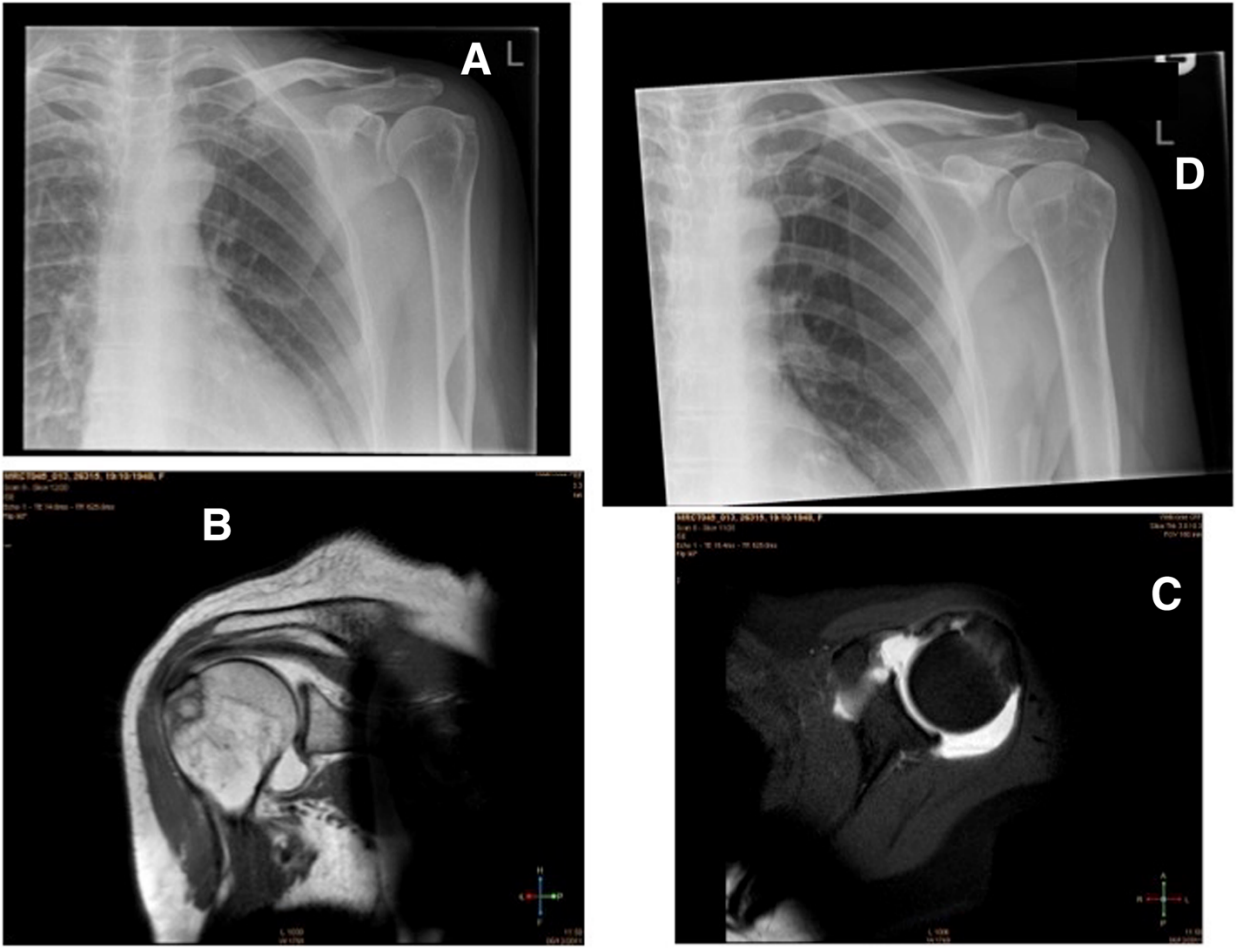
a Left shoulder Plain radiograph coronal plane reported as ‘normal’. b Coronal plain MR scan and (c) MR arthrogram both showing occult fracture greater tuberosity. d Plain radiograph coronal plain X-ray taken 2 weeks later showing undisplaced fracture greater tuberosity
